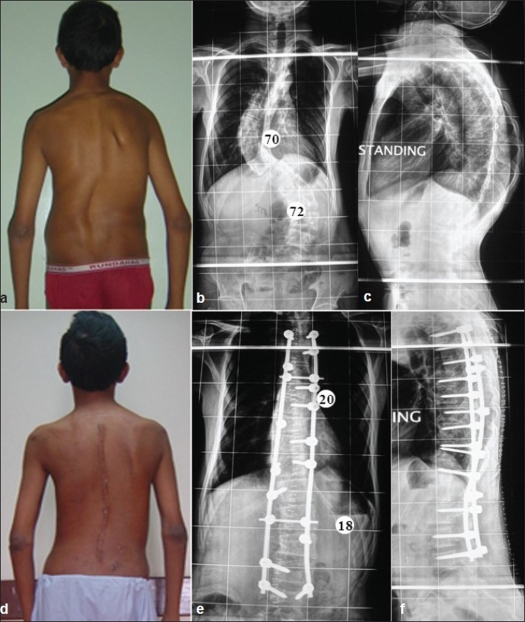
Clinical picture (a) and whole spine anteroposterior (b) and lateral (c) radiographs showing typical King Type I curve with Cobb's angle of 70° (thoracic) and 72° (Lumbar). Follow up clinical picture (d) and radiograph anteroposterior (e) and lateral (f) showing good correction and reduction of Cobb's angle to 20° (thoracic) and 18° (Lumbar)
